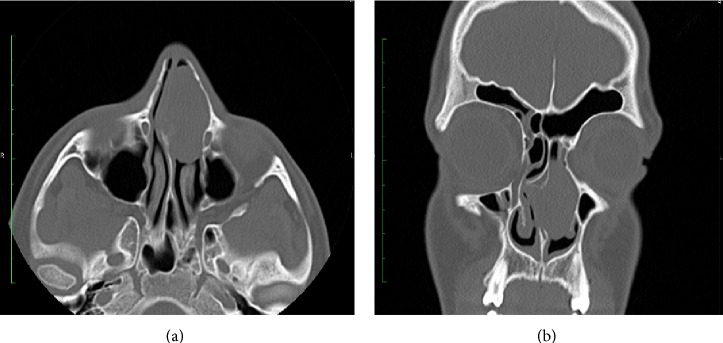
The image of the mass at the left nasal cavity in axial (a) and coronal (b) paranasal CT.
